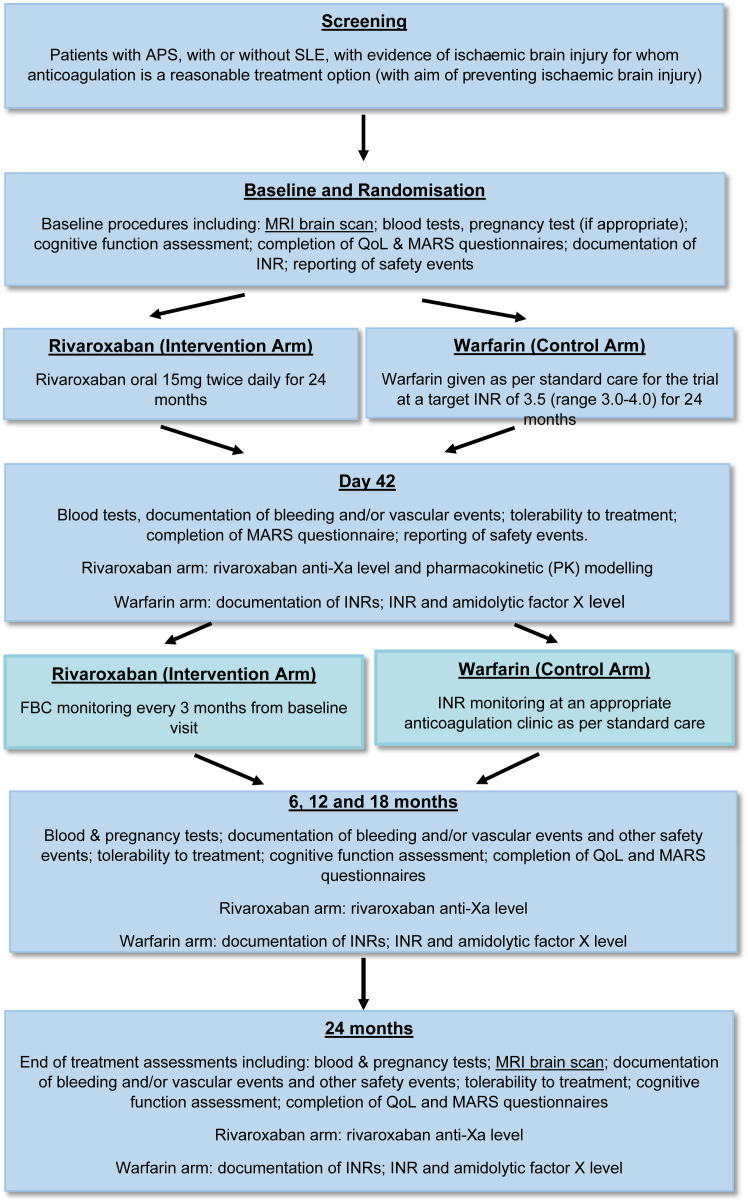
Overview of the trial schedule. APS, antiphospholipid syndrome; FBC, full blood count; INR, international normalized ratio; MARS, Medication Adherence Rating Scale; MRI, magnetic resonance imaging; QoL, quality of life; SLE, systemic lupus erythematosus.
